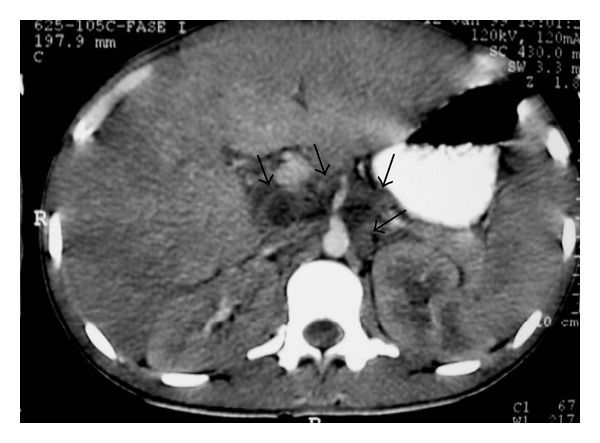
Nodal disease. Axial contrast-enhanced CT shows retroperitoneal lymph nodes (arrows).
